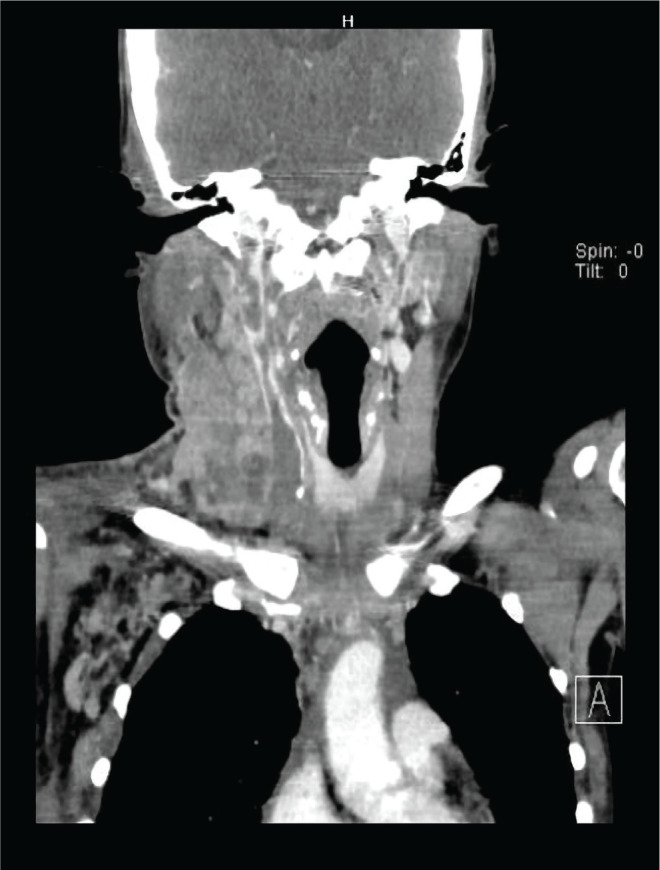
CT scan of the neck (coronal view) showing extensive thrombosis of the right internal jugular vein.
